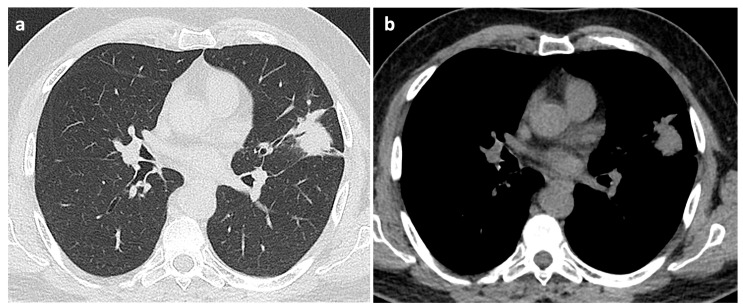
Parenchymal (a) and mediastinal (b) windows of axial thin-section unenhanced chest CT scans of a 54-year-old man with AFOP. Images show pseudonodular consolidation in the left upper lobe adjacent to the left fissure, with thin air bronchogram and a neighboring micronodule.
